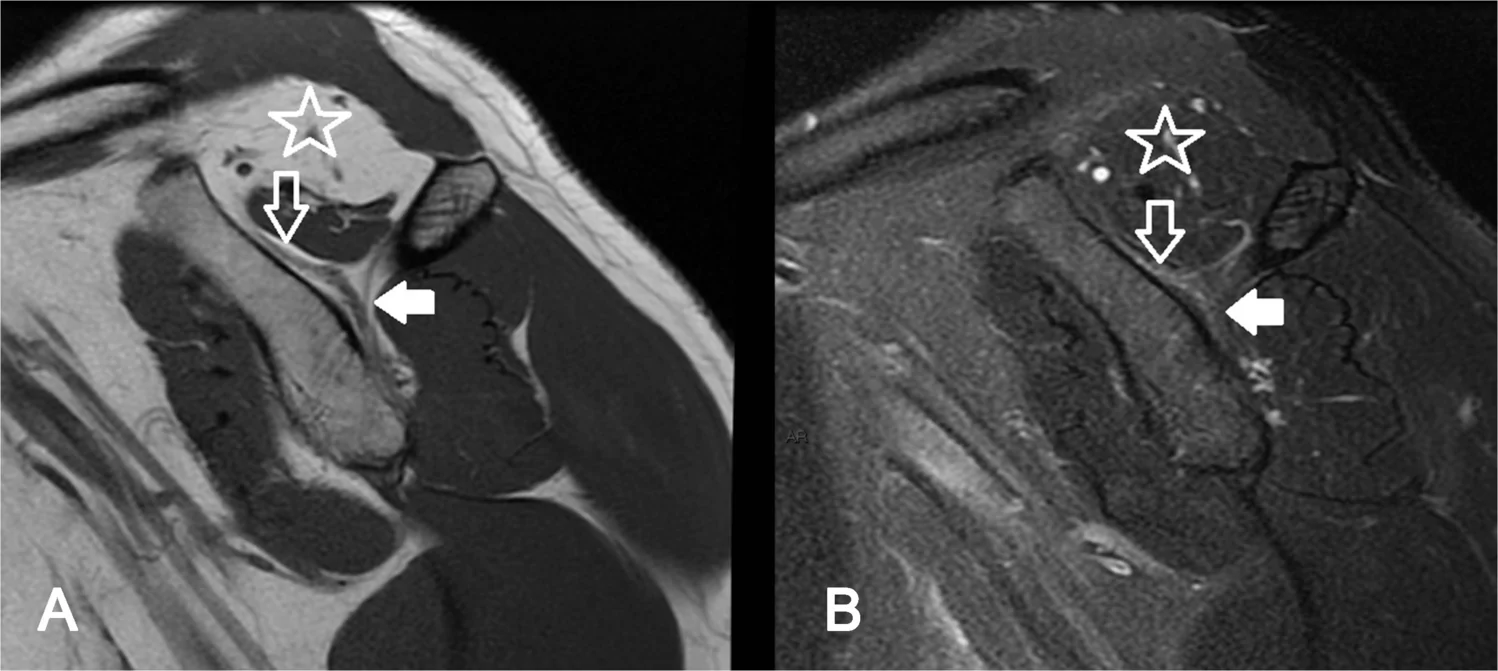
MR of the Shoulder at the Suprascapular and Spinoglenoid notch. Sagittal oblique T1-weighted image (A) and corresponding T2 with Fat Saturation (B) displays the nerve with normal morphology and signal, without intrinsic or extrinsic mass, as it courses through the level of the suprascapular notch (white open arrow) and its distal passage and descent through the level of the spinoglenoid notch (white solid arrow). Severe atrophy and decreased muscle volume of the supraspinatus muscle are documented (white open star)
